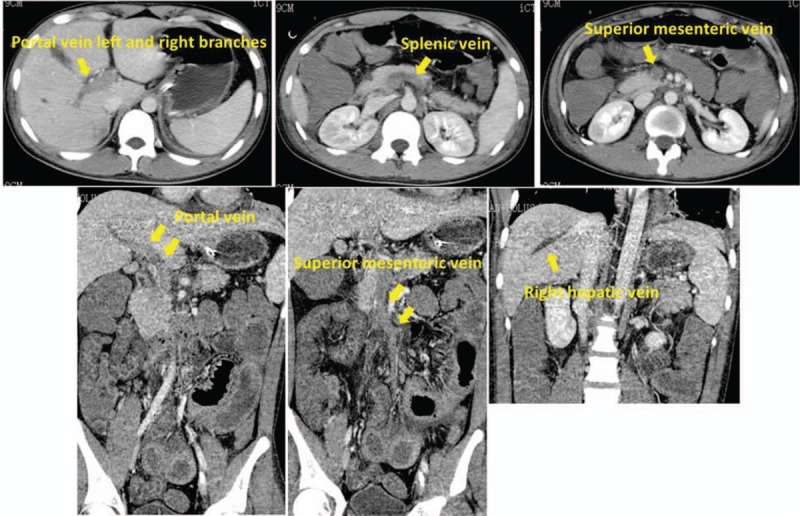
Whole abdominal enhanced computed tomography scan on July 25, 2017. Both axial and reconstructed images showed extensive venous filling defects, suggesting extensive venous thrombosis that was not significantly improved since July 21. The thrombosis was accompanied by partial small intestine expansion and intestinal wall edema.
